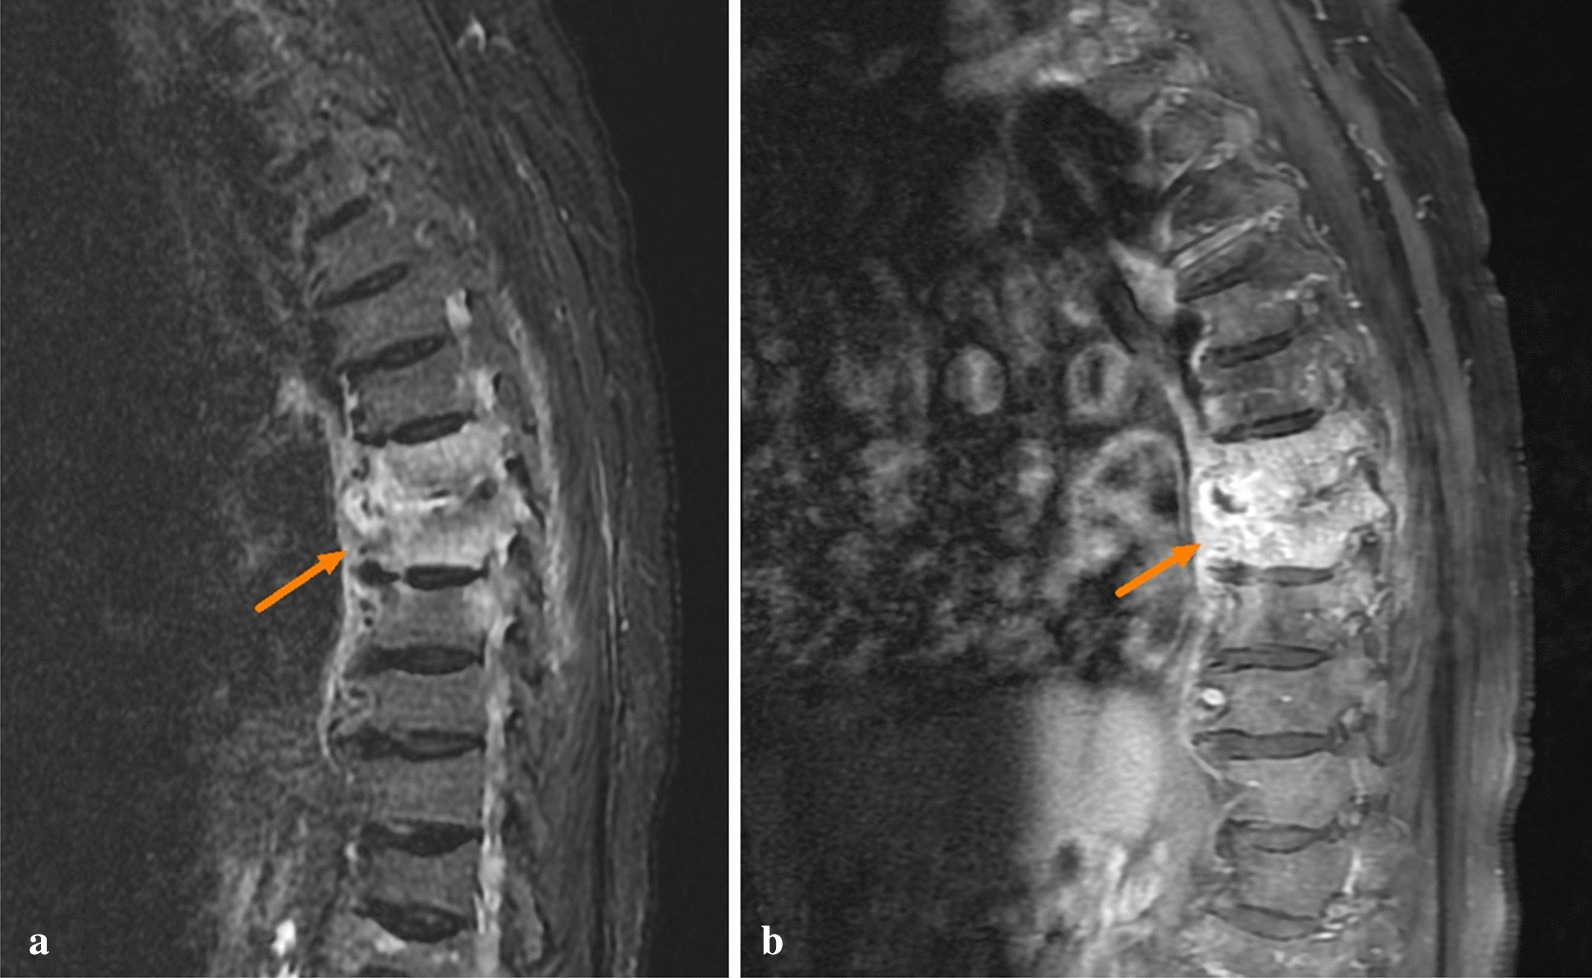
Magnetic resonance imaging of short T1 inversion recovery sequence (a) and contrast-enhanced T1 sequence (b). Enhanced signals between the seventh and eighth thoracic vertebra segments (arrows )
